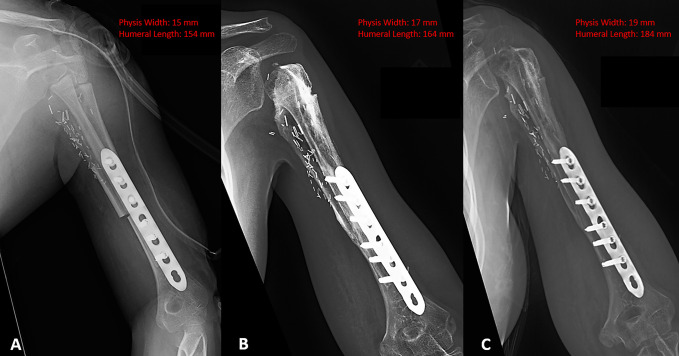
Immediate postoperative magnification correction radiographs showing a physis width of 15 mm and a humeral length of 154 mm (A) as measured from the top of physis to the distal olecranon fossa. At 15-month postoperative, the allograft/host junction had healed and the physis became spherical, with a width of 17 mm and humeral length of 164 mm (B), and at 27 months postoperative, the width was 19 mm with a humeral length of 184 mm (C).
